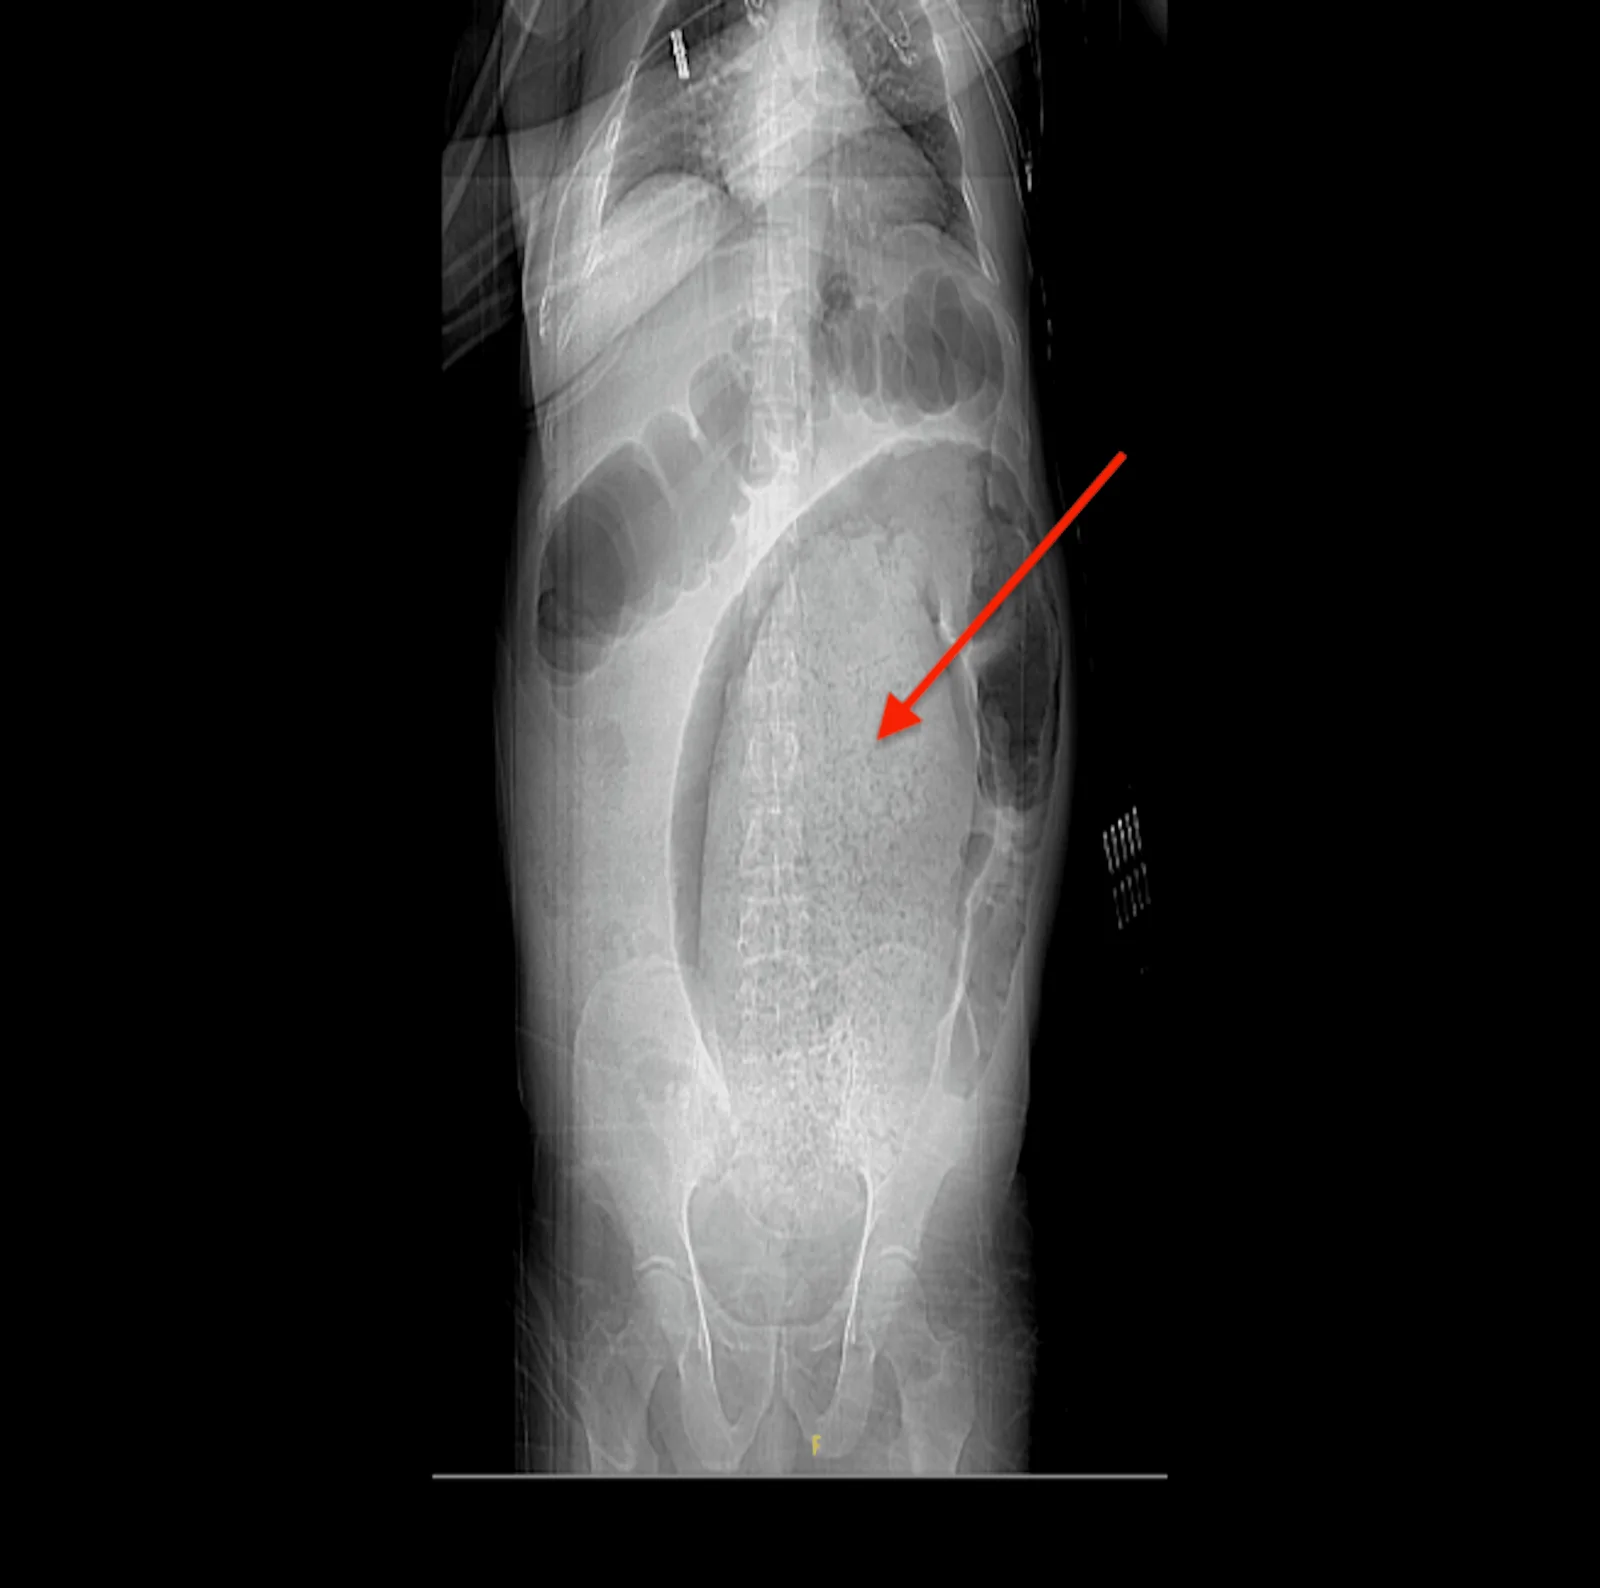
Plain X-ray abdomen Plain abdominal radiograph demonstrating a giant rectosigmoid fecaloma (red arrow) with marked diffuse gaseous distension of the colon, consistent with colonic ileus and subacute large bowel obstruction.
